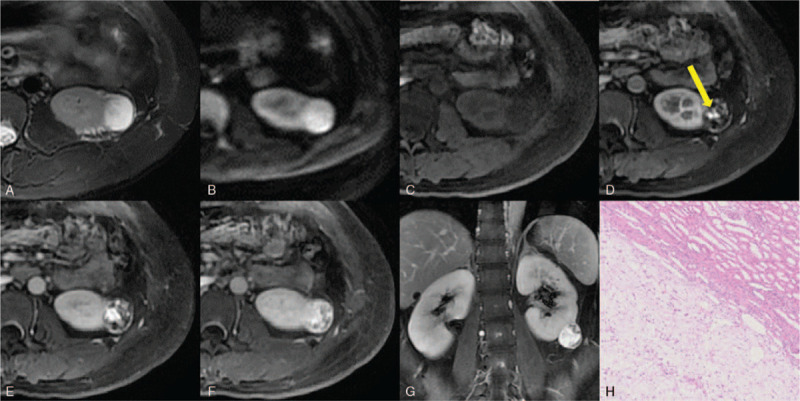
A 42-year-old woman with a 29 × 26 × 28 mm round mass in the left kidney diagnosed as hemangioblastoma. (A) Axial, fat-suppressed, T2-weighted images and (B) diffusion-weighted images showed significant hyperintensity of the mass. (C) In axial precontrast, fat-suppressed, T1-weighted images, the mass appeared hypointense compared with the renal parenchyma. (D) Axial corticomedullary phase fat-suppressed, gadolinium-enhanced, T1-weighted images showed peripheral nodular enhancement (yellow arrow). (E) Axial nephrographic phase, (F) axial delayed phase, and (G) coronal delayed phase fat-suppressed, gadolinium-enhanced, T1-weighted images showed progressive centripetal enhancement and almost complete “filling in” in the delayed phase. (H) The tumor was surrounded by a fibrous capsule and had a well-demarcated border against the surrounding renal parenchyma, HE × 100.
